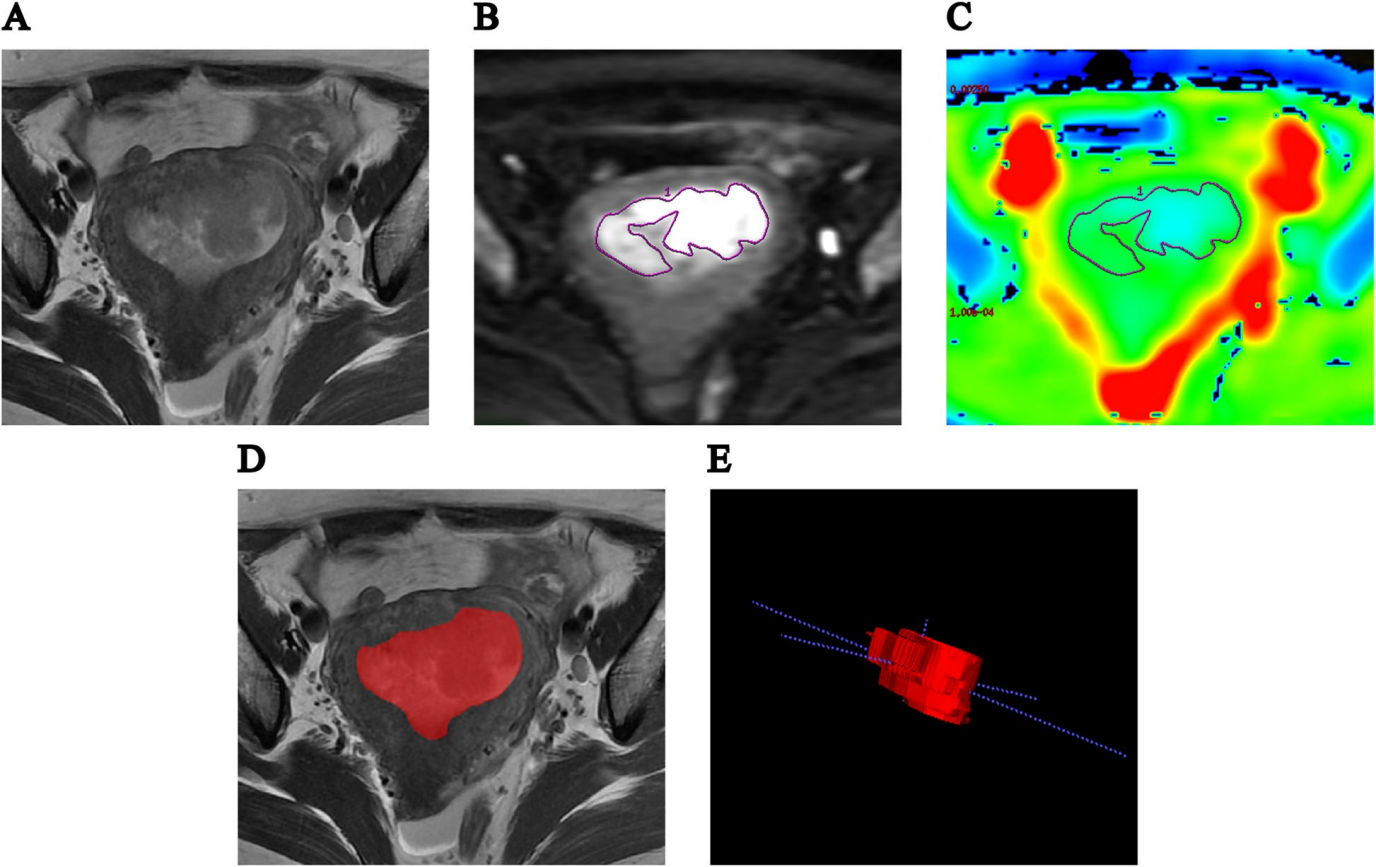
MR images of a 52-yearold woman with endometrioid carcinoma of stage IA. A Axial T2-weighted image demonstrated a mass in the uterine cavity, approximately 5.9 cm × 4.0 cm in size. B DWI (b = 1000 s/ mm^2^) showed the method of placing ROI within the tumor area. C ADC image showed that the ADC_mean_, ADC_min_ and ADC_max_ value were 0.710 × 10^− 3^, 0.673 × 10^− 3^ and 0.914 × 10^− 3^ mm^2^/s. D T2-weighted image showed the ROI of tumor segmentation. E The three-dimensional volume of interest
